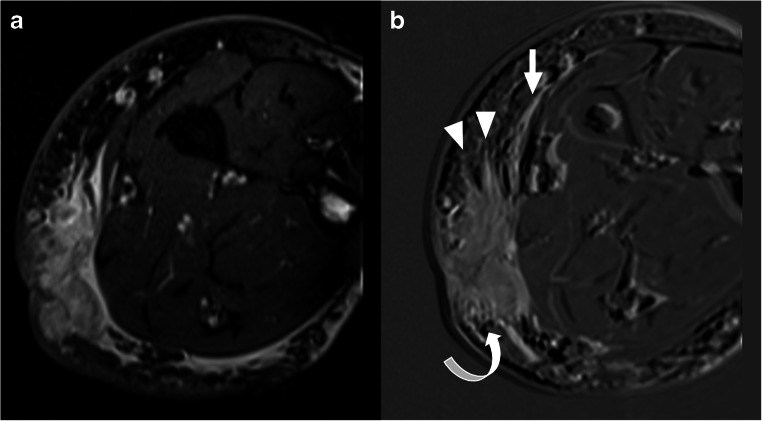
Axial T2-weighted (a) and subtraction gadolinium chelate-enhanced (b) images at the level of a forearm demonstrate a residual tumor (pathologically proven myxofibrosarcoma) after a Whoops procedure with unsharp tumor margins (curved arrow), tumor spiculae (arrowheads), and a tumor tail on the superficial muscle fascia (straight arrow)
